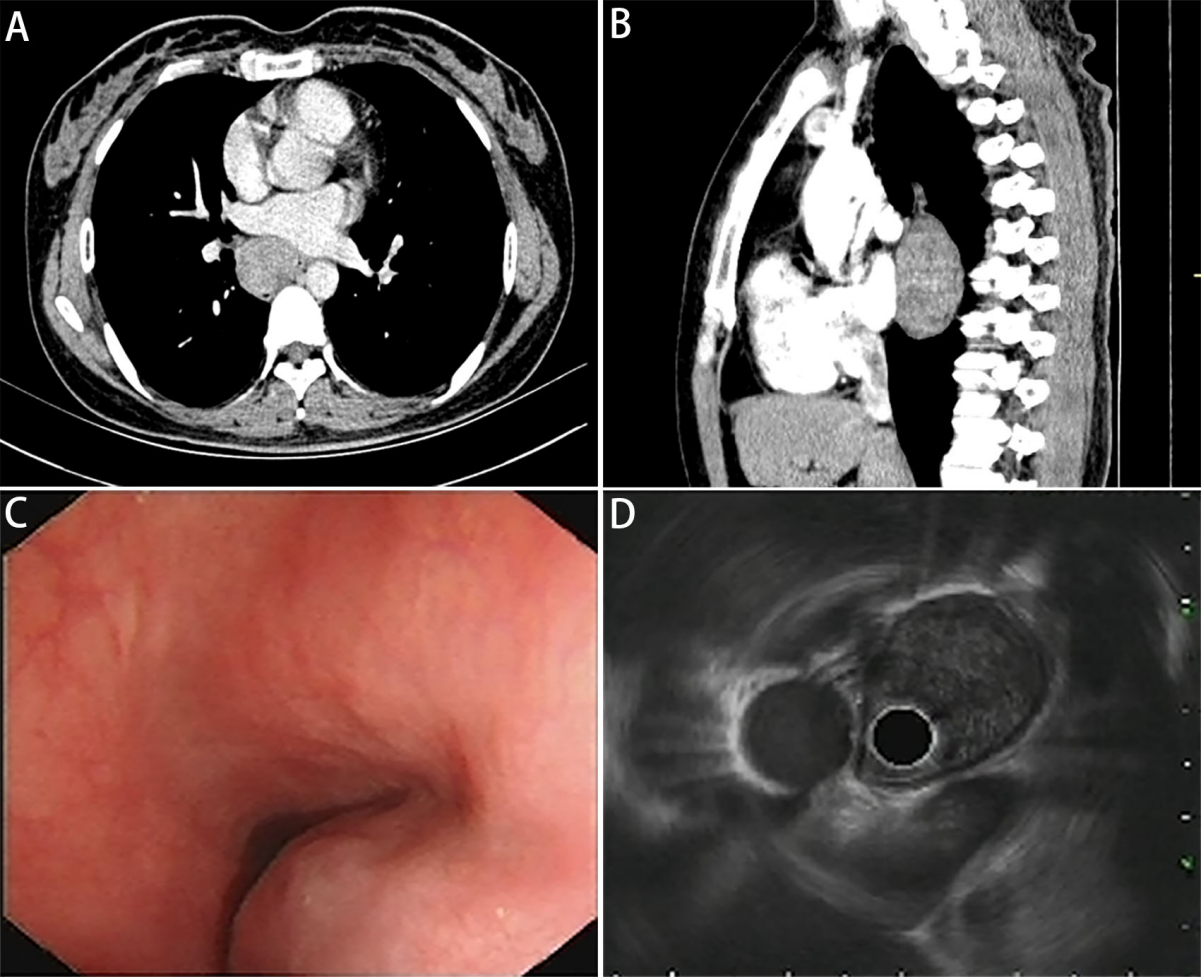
Images of pulmonary mediastinum CT and echogatstroscopy. (A) Transverse position and (B) sagittal position of pulmonary mediastinum CT show a mass located in the right posterior mediastinum approximately 37 × 28 × 67 mm in size, and the boundary with the adjacent esophagus is unclear. (C) The white light endoscopy view and (D) endoscopic ultrasound image show a 34.5 mm × 32.6 mm semispherical uplift with smooth surface mucosa and intraluminal growth pattern originating from the esophageal submucosal layer, with uniform hypoechoic change and a clear boundary.
